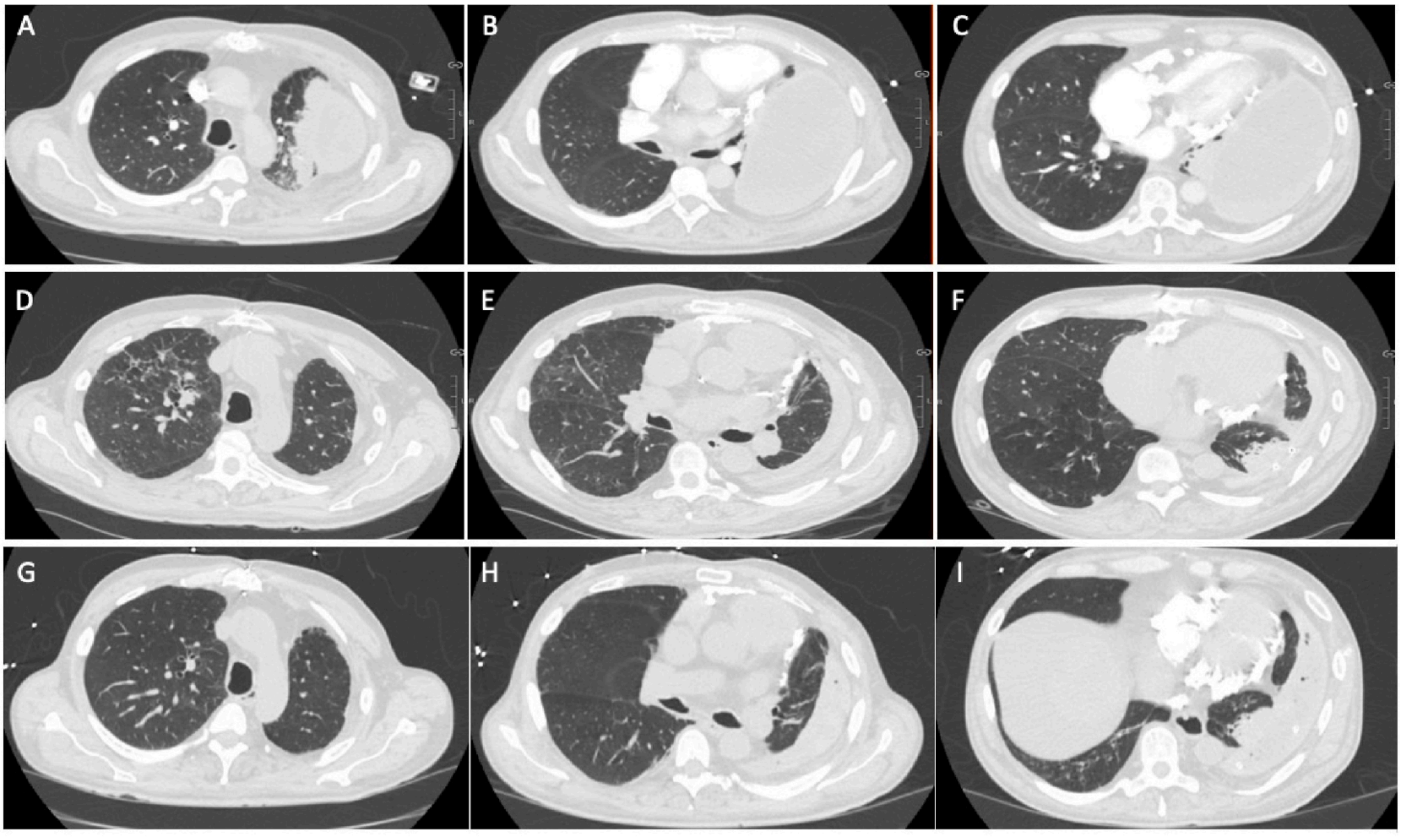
A–C: Chest CT scan on initial referral to our pleural clinic, demonstrating a large loculated effusion. D-F Chest CT scan four-months following tunneled pleural catheter placement demonstrating pleural symphysis. G-I Most recent chest CT scan available, 17-months following TPC placement, showing pleural symphysis with some associated rounded atelectasis.
